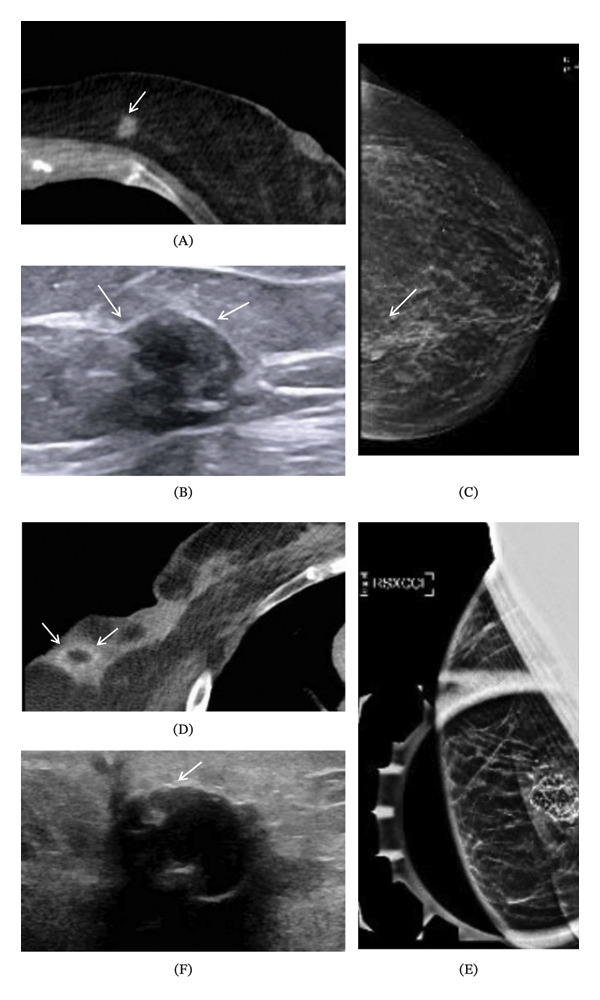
Benign findings incidentally noted on chest CT. (A) Chest CT on soft tissue window demonstrating soft tissue density oval mass in the posterior inner left breast (arrow). (B) Grayscale ultrasound demonstrating oval mass with heterogeneous echotexture and well‐circumscribed margins which was felt to likely correlate to the CT finding (arrows). This mass was subsequently biopsied and found to be consistent with fibroadenoma. (C) Mammogram of the left breast in the craniocaudal (CC) view demonstrates an oval mass with high density and circumscribed margins at the 9 o’clock position (arrow). (D) Chest CT on soft tissue window demonstrating a rim‐enhancing oval mass in the right lateral breast, closely associated with postsurgical changes of prior mastectomy. (E) Right laterally exaggerated craniocaudal (XCCL) spot compression view demonstrates a fat‐containing, round mass with circumscribed margins and associated dystrophic calcifications at the 9 o’clock position in the right lateral breast. (F) Grayscale ultrasound demonstrating mass closely associated with the mastectomy scar with echogenic rim (white arrow) and associated microcalcifications (arrowhead) and posterior shadowing, consistent with postsurgical fat necrosis.
